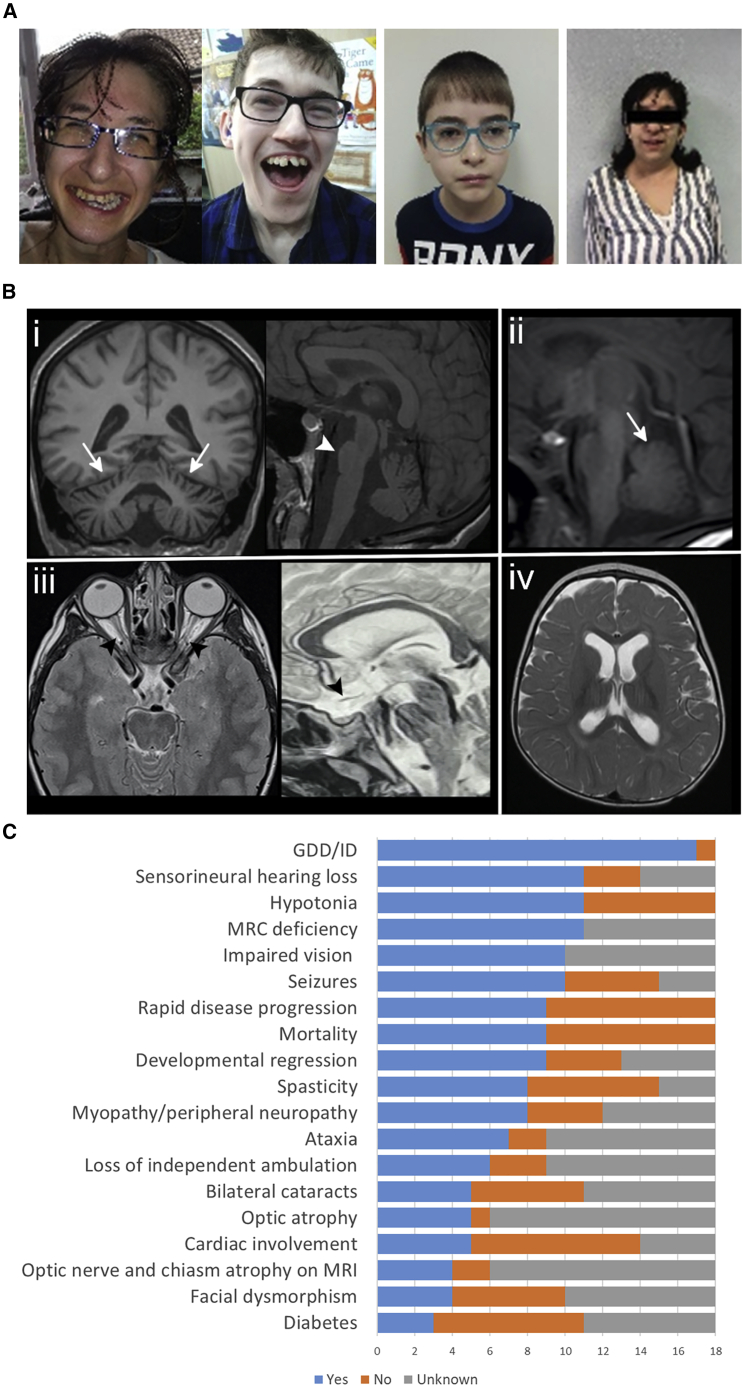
Clinical features and neuroimaging findings of the individuals with bi-allelic *LETM1* variants (A) From left to right, facial photos of the affected individuals F1:S1, F1:S2, F5:S1, and F8:S1. All persons wear glasses due to bilateral optic atrophy. All persons have prominent noses. F1:S1 and F1:S2 show long thin faces, low-set ears, and teeth abnormalities. (B) In (i) (F1:S1), severe cerebellar atrophy (arrows) and pontine hypoplasia (arrowheads) are shown, while in (ii) (F6:S1), only mild vermian hypoplasia is noted. In (iii) arrowheads point at the severe optic nerve and chiasm atrophy in 2 different individuals (F5:S1 and F7:S2). Mild ventricular dilatation is present in (iv) (F3:S1). (C) Clinical features of the affected individuals with bi-allelic *LETM1* variants. GDD, global developmental delay; ID, intellectual disability; MRI, magnetic resonance imaging; MRC, mitochondrial respiratory chain.
